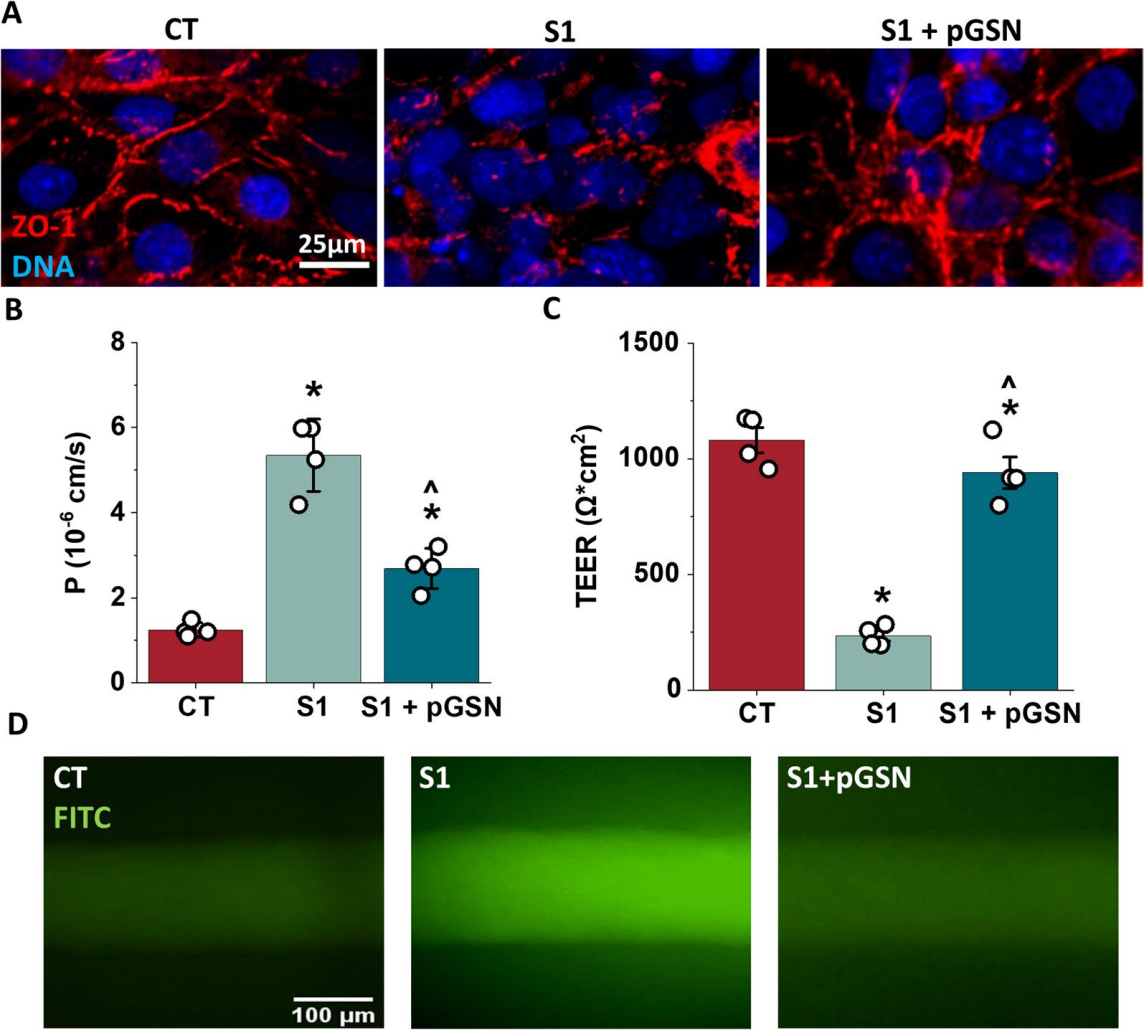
Plasma gelsolin reverses the destructive effect of SARS-CoV-2 Spike protein S1 subunit on blood–brain barrier function in the 3D flow model. Confocal images of hCMEC/D3 cells (panel A), tight junction protein ZO-1 (red), and nuclei (blue). Permeability coefficient measured from dextran experiments for endothelial channels exposed to S1 and S1 + pGSN (Panel B). TEER measurement results (Panel C). Images demonstrating the measurement of vessel permeability using 4 kDa FITC-dextran (green) and the effects of the S1 and S1 + pGSN (Panel D). Barrier permeability and TEER measurement were performed after 4 h of perfusion with 10 nM of S1 and S1 + pGSN. The data represent the mean ± SEM of four independent experiments (*n* = 4). * and ^ indicate statistical significance at *p* ≤ 0.05 compared to CT and S1, respectively, by one-way ANOVA and Tukey post hoc test
